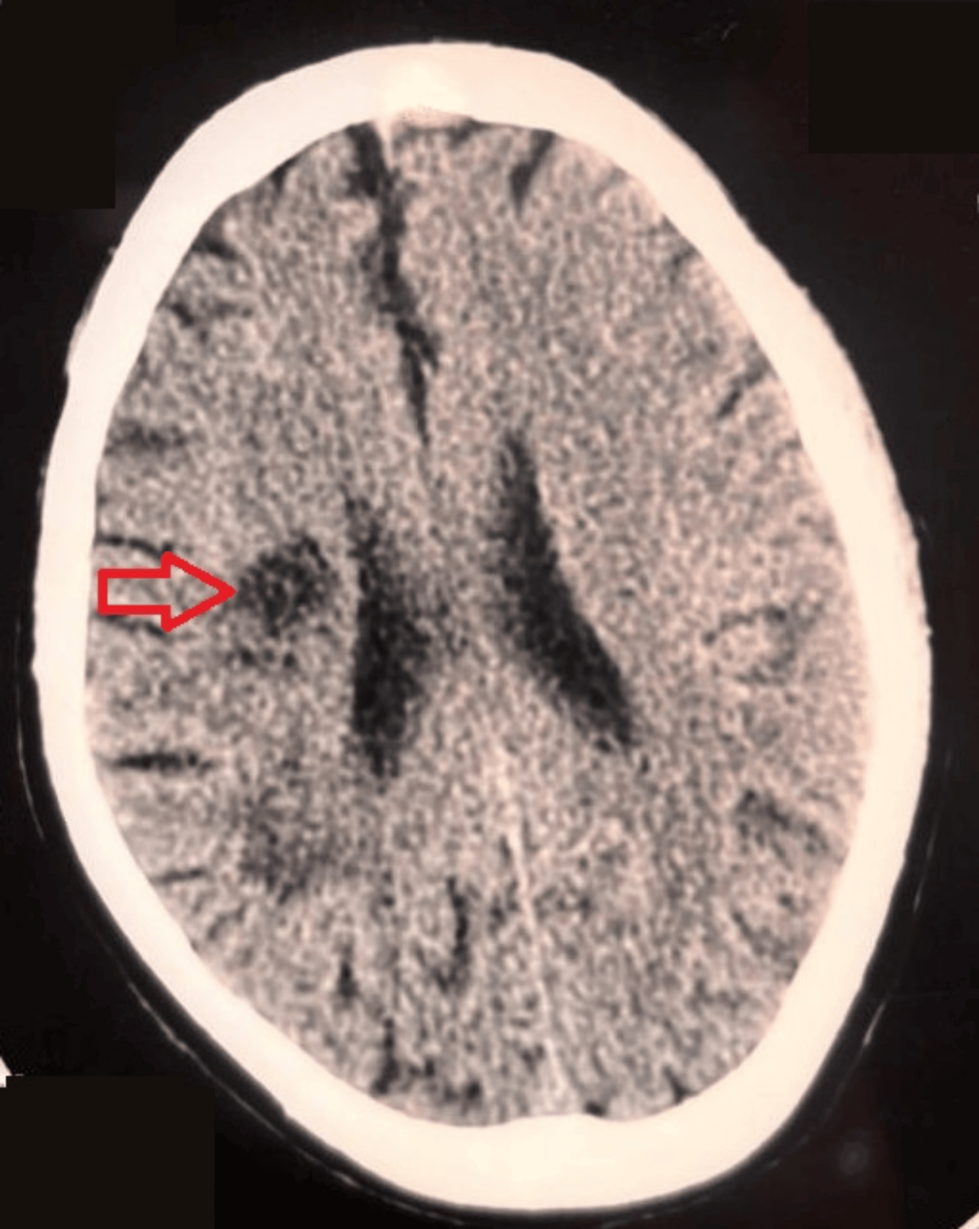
Non-contrast CT scan of the brain showing a lacunar infarct (arrow)
